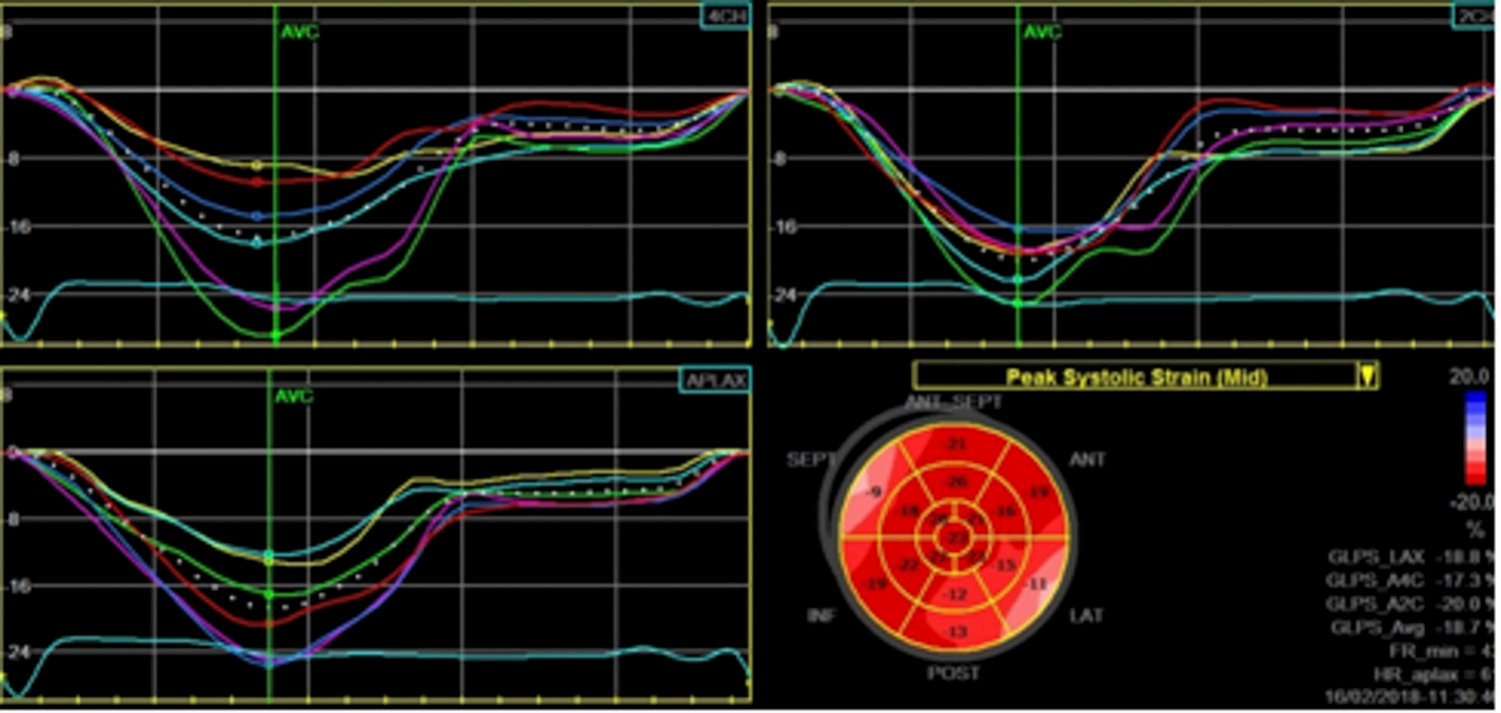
Left ventricular strain curves and bull’s eye plots. LV longitudinal strain curves derived from speckle-tracking analysis for each LV segment at apical 4-chamber view (left panel), paraesternal long-axis view (left lower panel), 2-chamber view (right upper panel), and bull’s eye” plot (right lower panel).
